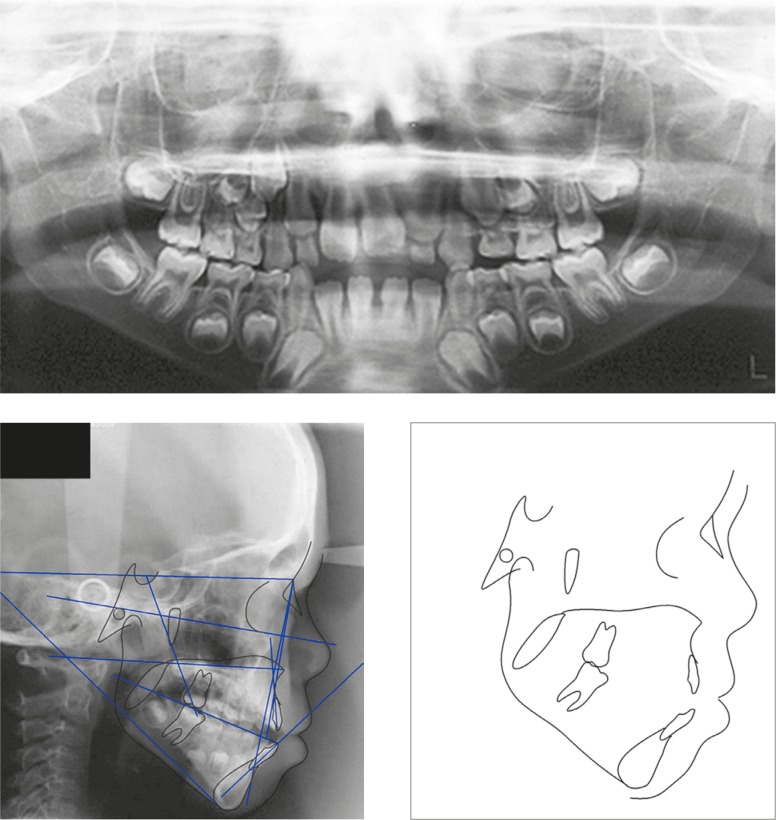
Panoramic radiograph and lateral cephalogram before treatment.
